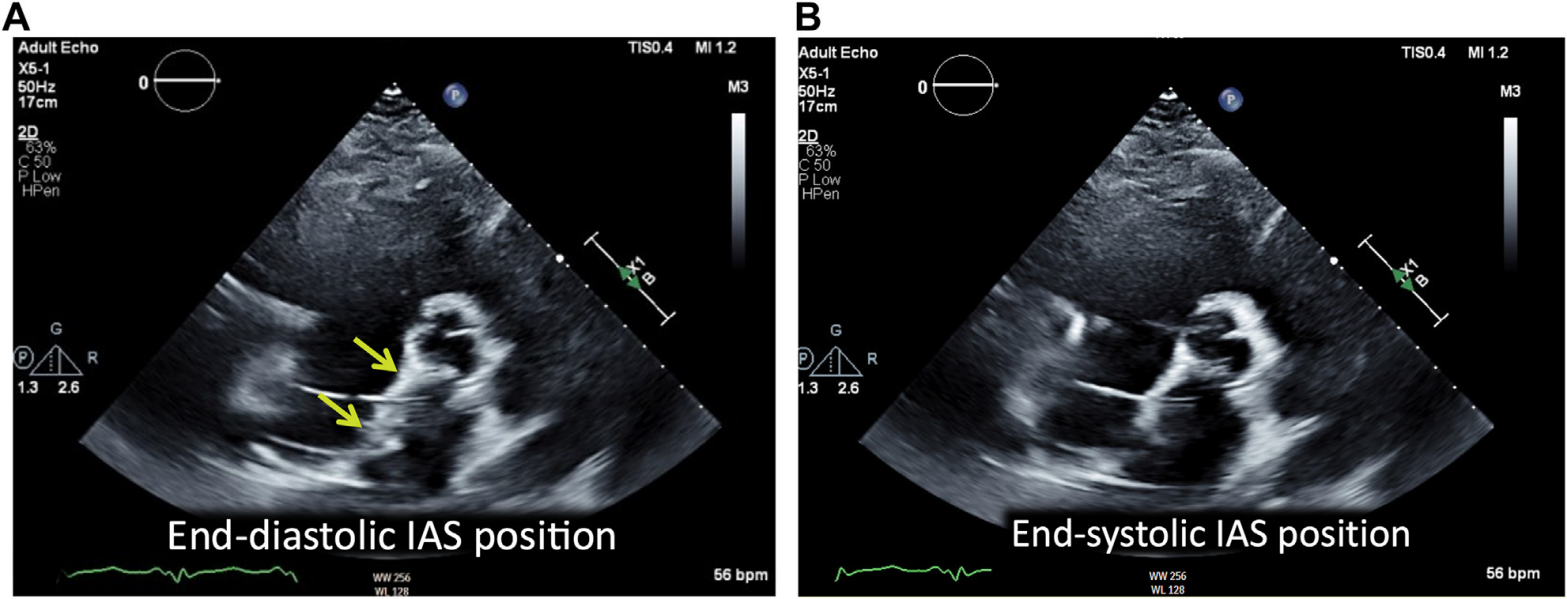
Two-dimensional TTE, basal parasternal short-axis diastolic (A) and systolic (B) views at admission, demonstrates that the leftward-shifted interatrial septum (IAS) is near midline at end-systole, suggestive of increased right atrial pressure.
